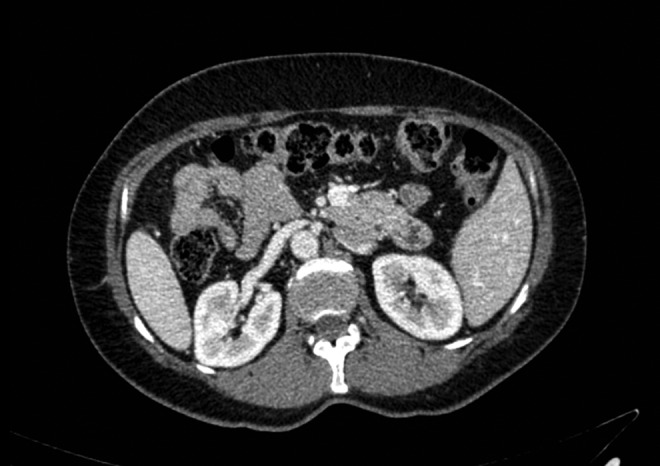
Preoperative donor CT angiogram shows longer right renal vein.
